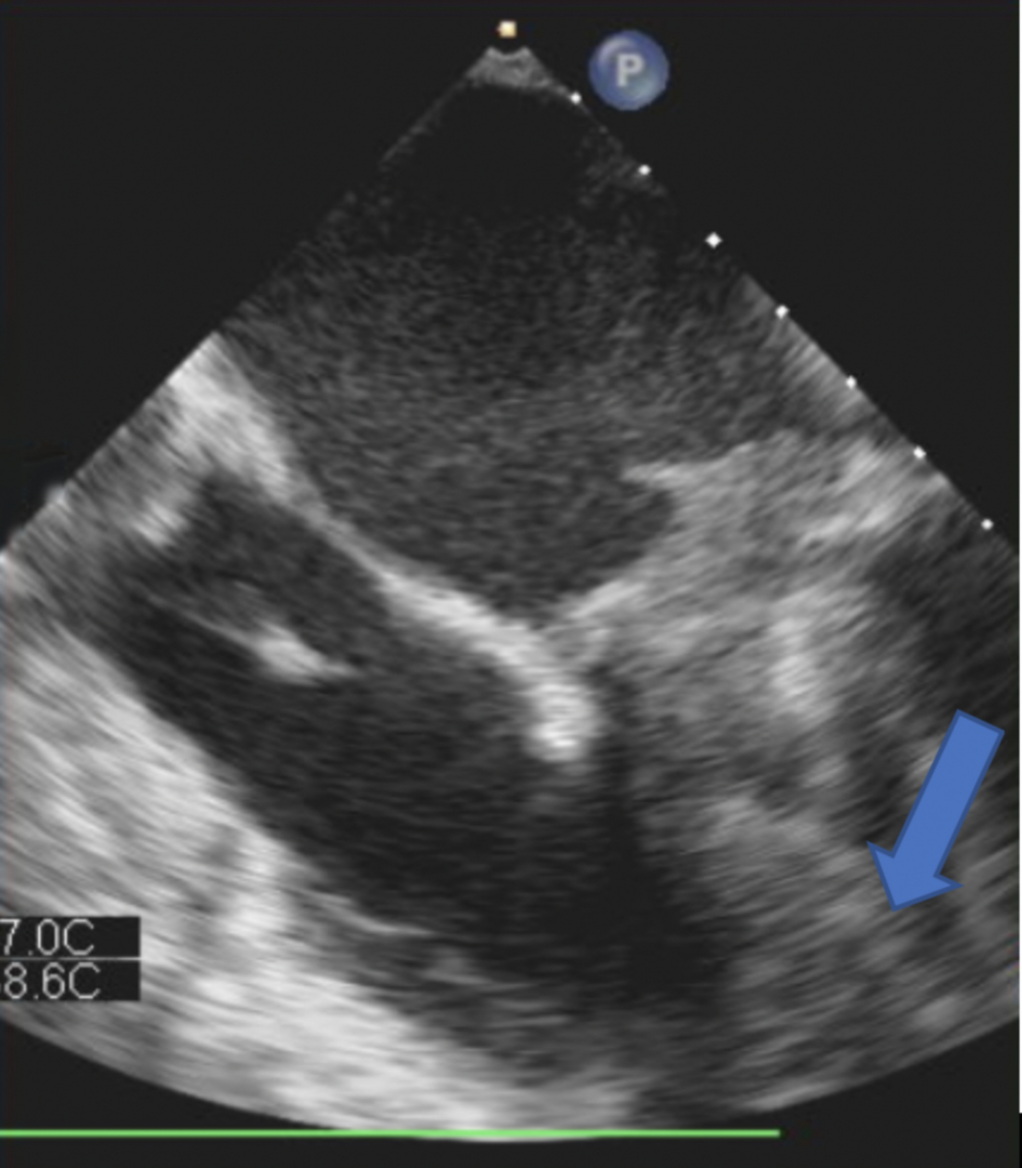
X-plane image of transesophageal echocardiography showing thrombus (Arrow) caked onto the mechanical mitral valve
